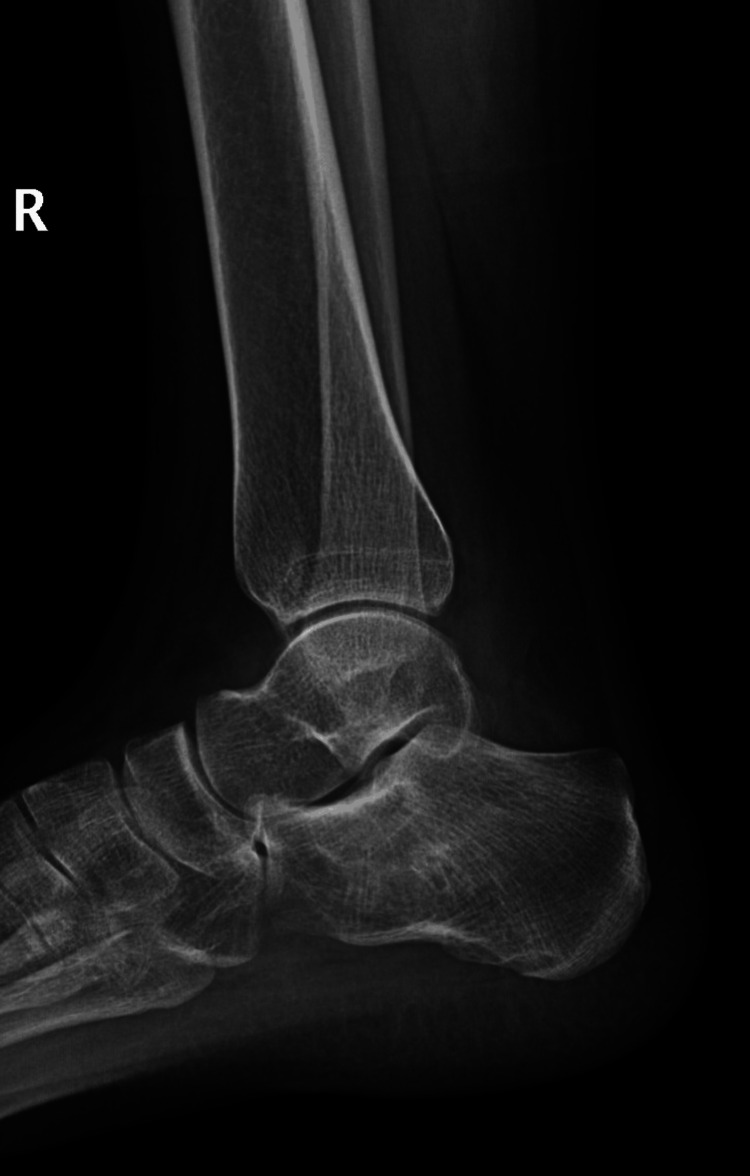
Lateral view X-ray of the right ankle at the time of presentation
